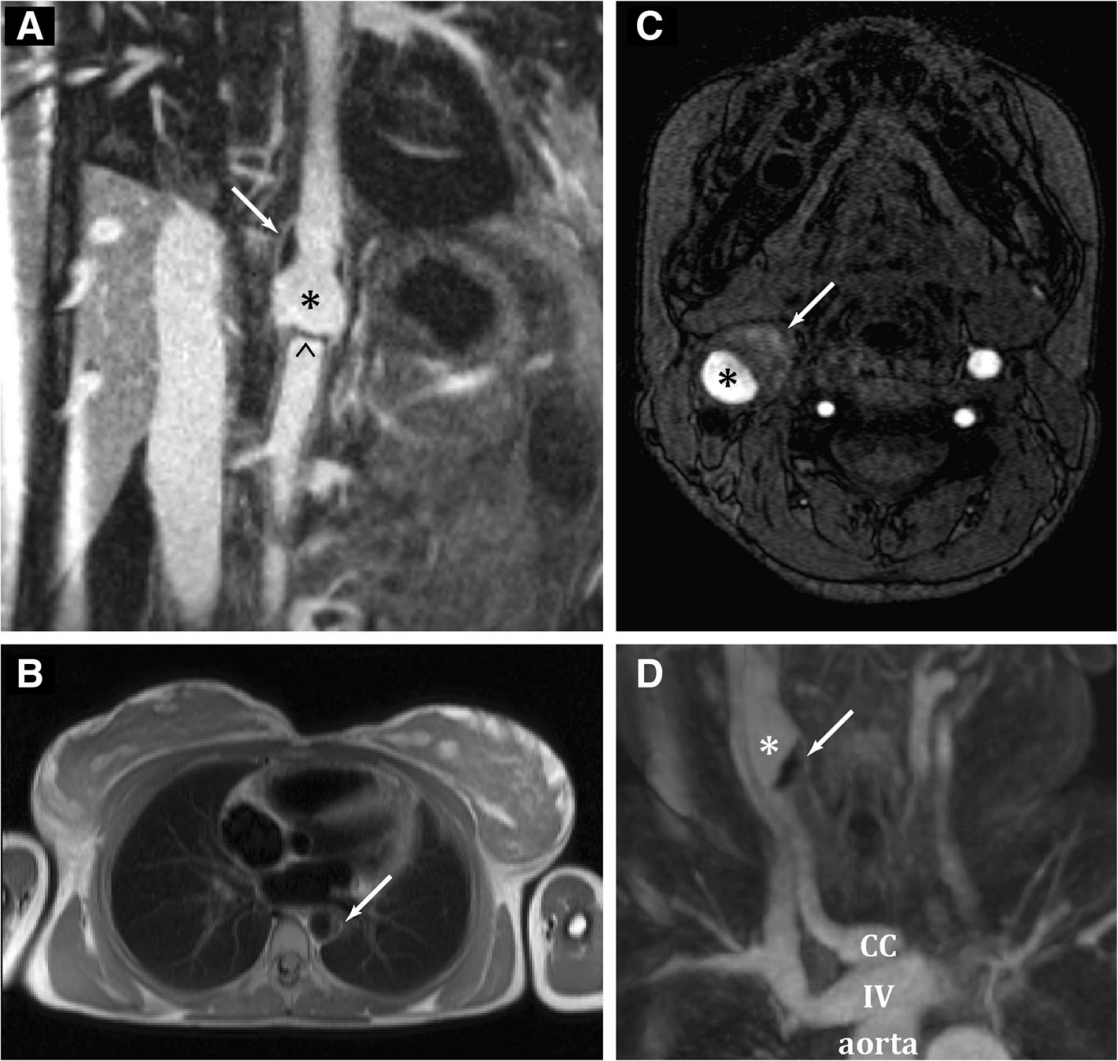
Vascular imaging in the three children with Takayasu arteritis and dissection. Legend: Patient 1: a A coronal reformat of the 3D volume rendered MRA dataset demonstrates the intramural hematoma (arrow) as well as a dissection flap (arrowhead) at the caudal end of the aneurysm (*) in the abdominal aorta. The intramural hematoma (arrow) is located at the proximal end of the aneurysm. The celiac axis arises immediately distal to the dissection flap with a stenotic origin. There is thickening of the aortic wall and a long-segment narrowing of the infra-renal aorta. Patient 2: b A T2 weighted turbo spin echo magnetic resonance image in the axial orientation shows a dissection flap in the thoracic descending aorta at the level of the left atrium (arrow). Patient 3: c The T1 weighted turbo spin echo and d the para-coronal reformat of a contrast-enhanced magnetic resonance angiogram show an aneurysm of the right carotid artery (*) with intramural hematoma (arrow). CC = Common carotid artery, IV = innominate vein
